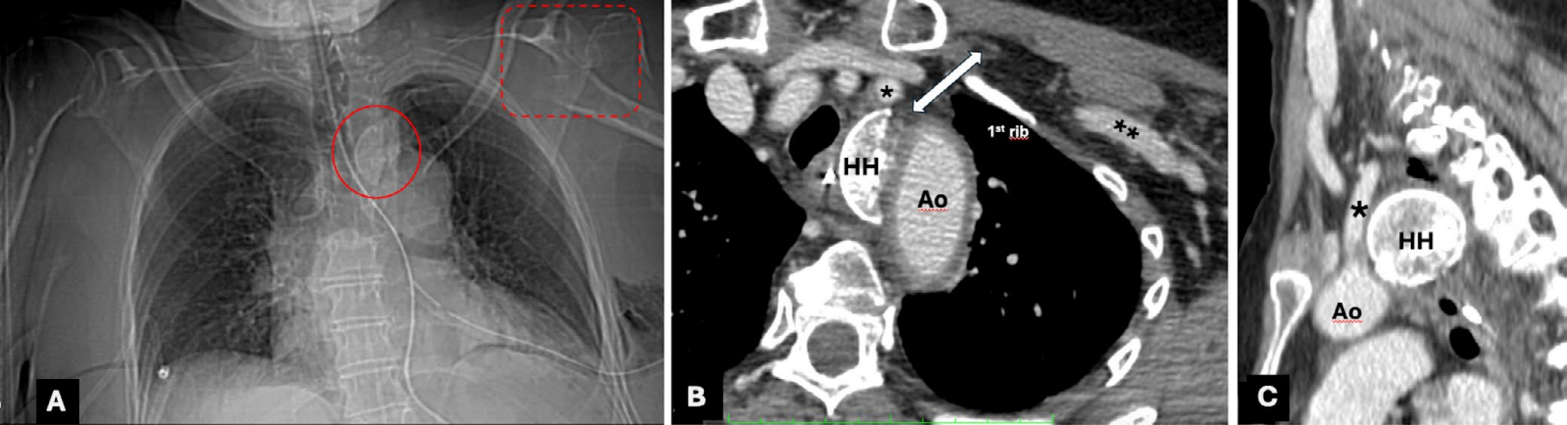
Chest x‐ray (A): Intramediastinal dislocation of humeral head (red circle) after its post‐traumatic fracture (box red dotted). Axial CT chest scan (B): Intramediastinal position of the humeral head, medially to the aortic arch (Ao), posteriorly to the left subclavian artery (asterisk), and laterally to the trachea. The bidirectional white arrow indicates the entry route into the mediastinum. Subclavian artery (double asterisk). Coronal CT chest scan (C): The bone fragment (HH) in the angle formed by the aortic arch (Ao) and the origin of the left subclavian artery (asterisk).
